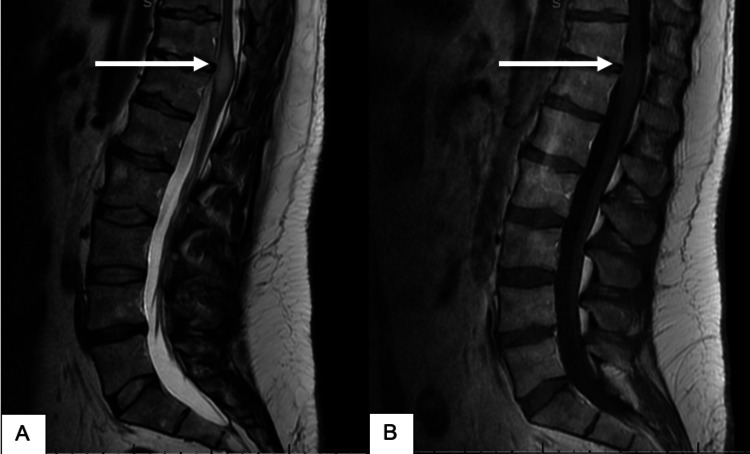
MRI of the lumbar spine pre-operatively, sagittal view (A) T2-weighted and (B) T1-weighted post-contrast images showing a non-enhancing intramedullary mass (white arrows) at the T11-12 level.
